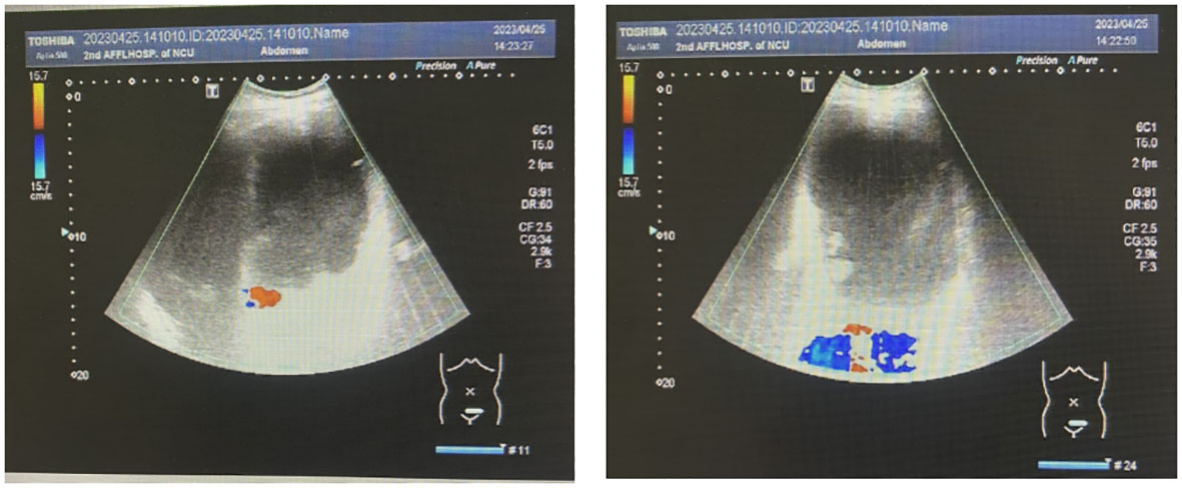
Abdominal color ultrasonography scans.
